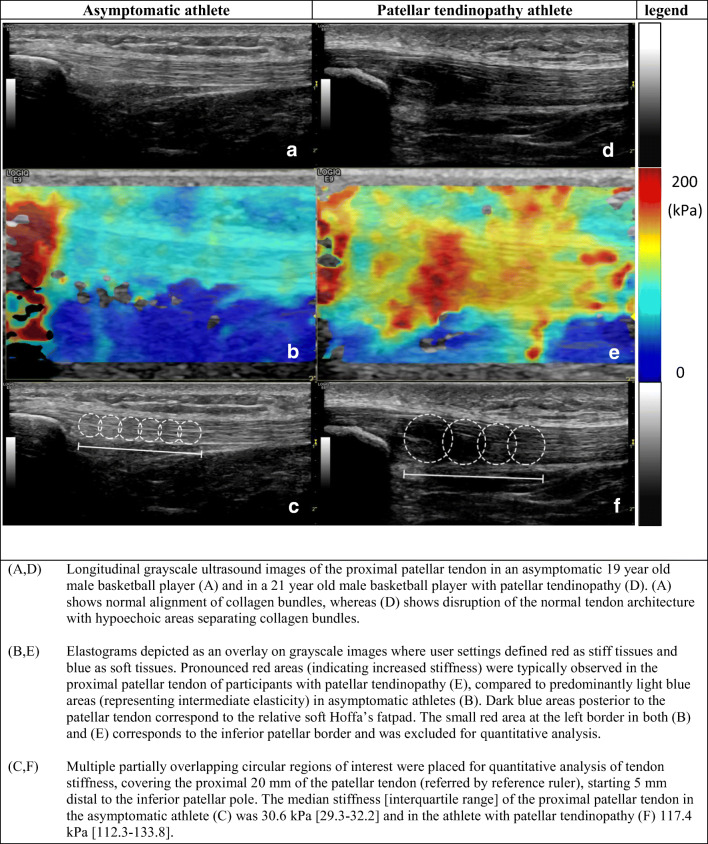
Grayscale US and corresponding shear-wave elastograms in an asymptomatic athlete and an athlete with patellar tendinopathy. a, d Longitudinal grayscale ultrasound images of the proximal patellar tendon in an asymptomatic 19-year-old male basketball player (a) and in a 21-year-old male basketball player with patellar tendinopathy (d). a shows normal alignment of collagen bundles, whereas d shows disruption of the normal tendon architecture with hypoechoic areas separating collagen bundles. b, e Elastograms depicted as an overlay on grayscale images where user settings defined red as stiff tissues and blue as soft tissues. Pronounced red areas (indicating increased stiffness) were typically observed in the proximal patellar tendon of participants with patellar tendinopathy (e), compared to predominantly light blue areas (representing intermediate elasticity) in asymptomatic athletes (b). Dark blue areas posterior to the patellar tendon correspond to the relative soft Hoffa’s fatpad. The small red area at the left border in both b and e corresponds to the inferior patellar border and was excluded for quantitative analysis. c, f Multiple partially overlapping circular regions of interest were placed for quantitative analysis of tendon stiffness, covering the proximal 20 mm of the patellar tendon (referred by reference ruler), starting 5 mm distal to the inferior patellar pole. The median stiffness (interquartile range) of the proximal patellar tendon in the asymptomatic athlete (c) was 30.6 kPa (29.3–32.2) and in the athlete with patellar tendinopathy (f) 117.4 kPa (112.3–133.8)
